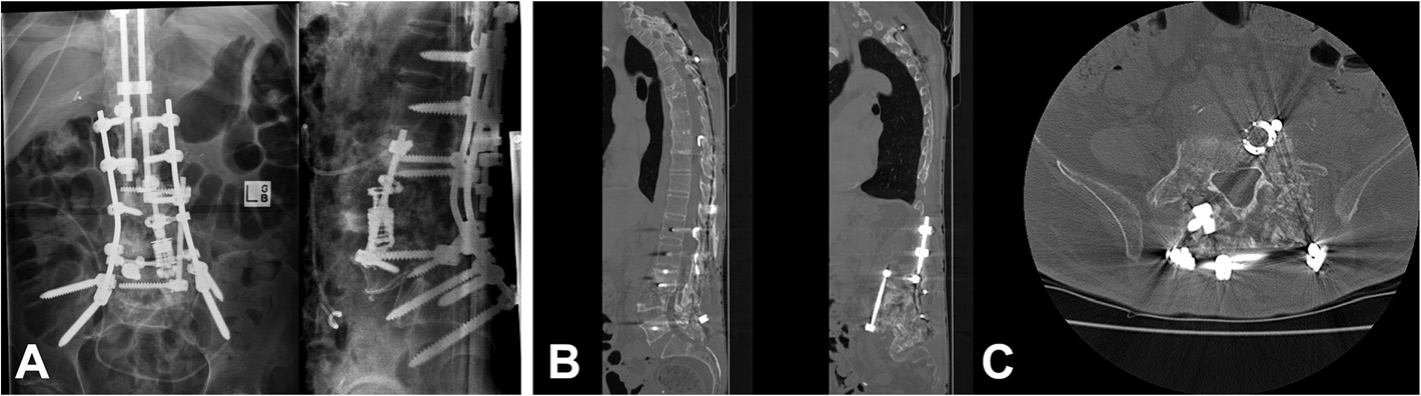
Plain radiographs and computed tomography scan showing revision and extension of posterior instrumentation to the ilium. (A) Anteroposterior and lateral radiographs showing revision and extension of posterior instrumentation to the ilium. (B) and (C) Sagittal and axial computed tomography scan taken after 6 weeks of immobilization following the revision surgery showing consolidation of the bone graft.
